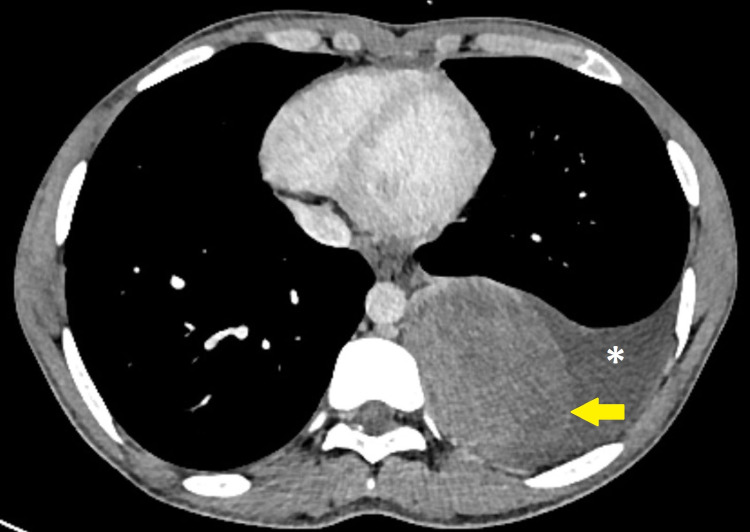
Axial section of contrast-enhanced CT chest shows mass lesion between the two layers of parietal pleura (yellow arrow) and moderate pleural effusion is seen (white asterisk)
